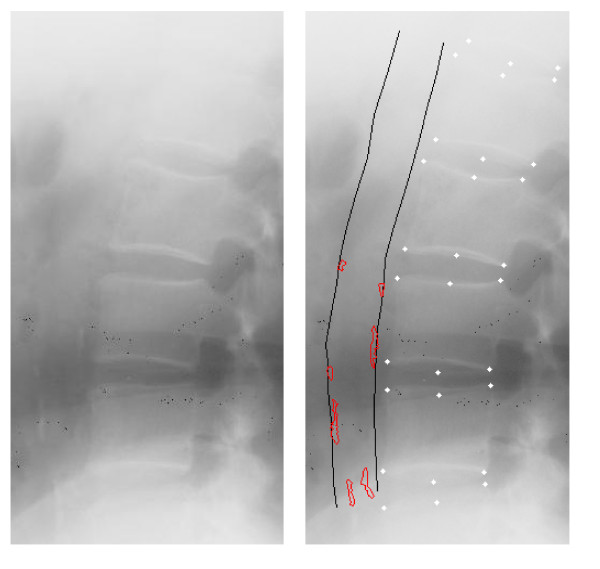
Calcification Annotations. Lateral lumbar X-ray with calcifications in the lower region without (a) and with (b) computer-mediated annotations performed by radiologist.
